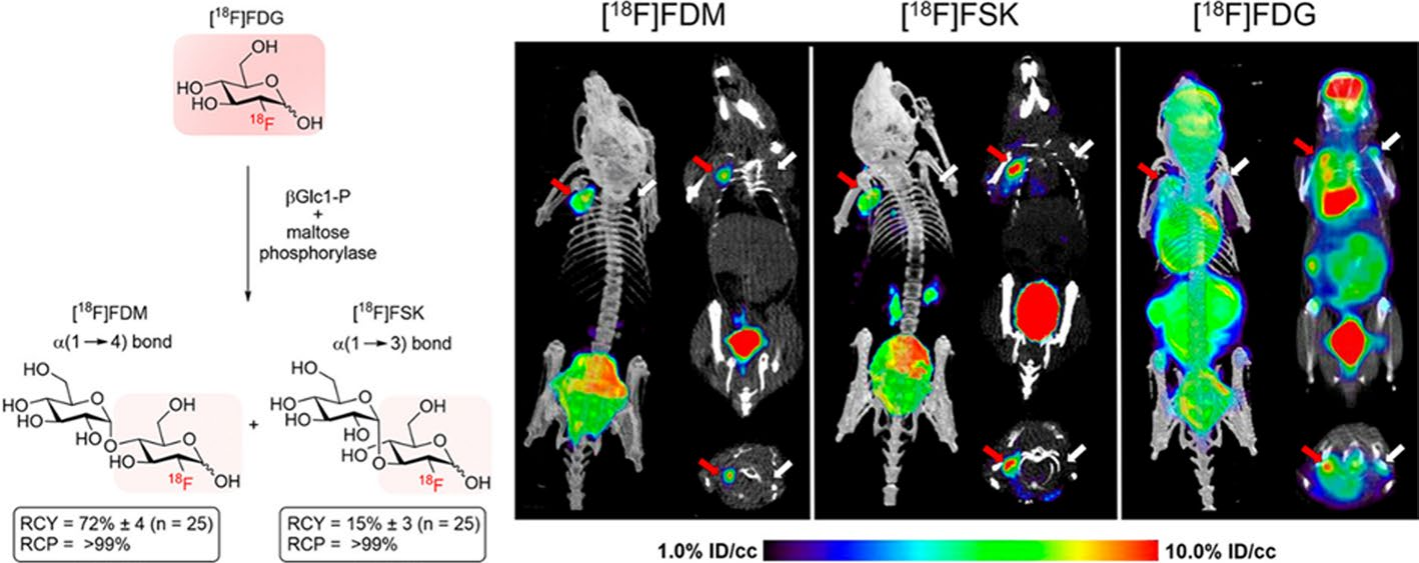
Chemoenzymatic, one step synthesis of [^18^F]FDM and [^18^F]FSK from [^18^F]FDG, and their selective uptake in an in vivo model of MRSA myositis (red arrow: site of inoculation with live bacteria; white arrow: site of inoculation with heat-killed bacteria). With permission from J Am Chem Soc 2023;145:17632–17642 (CC BY 4.0 License)
